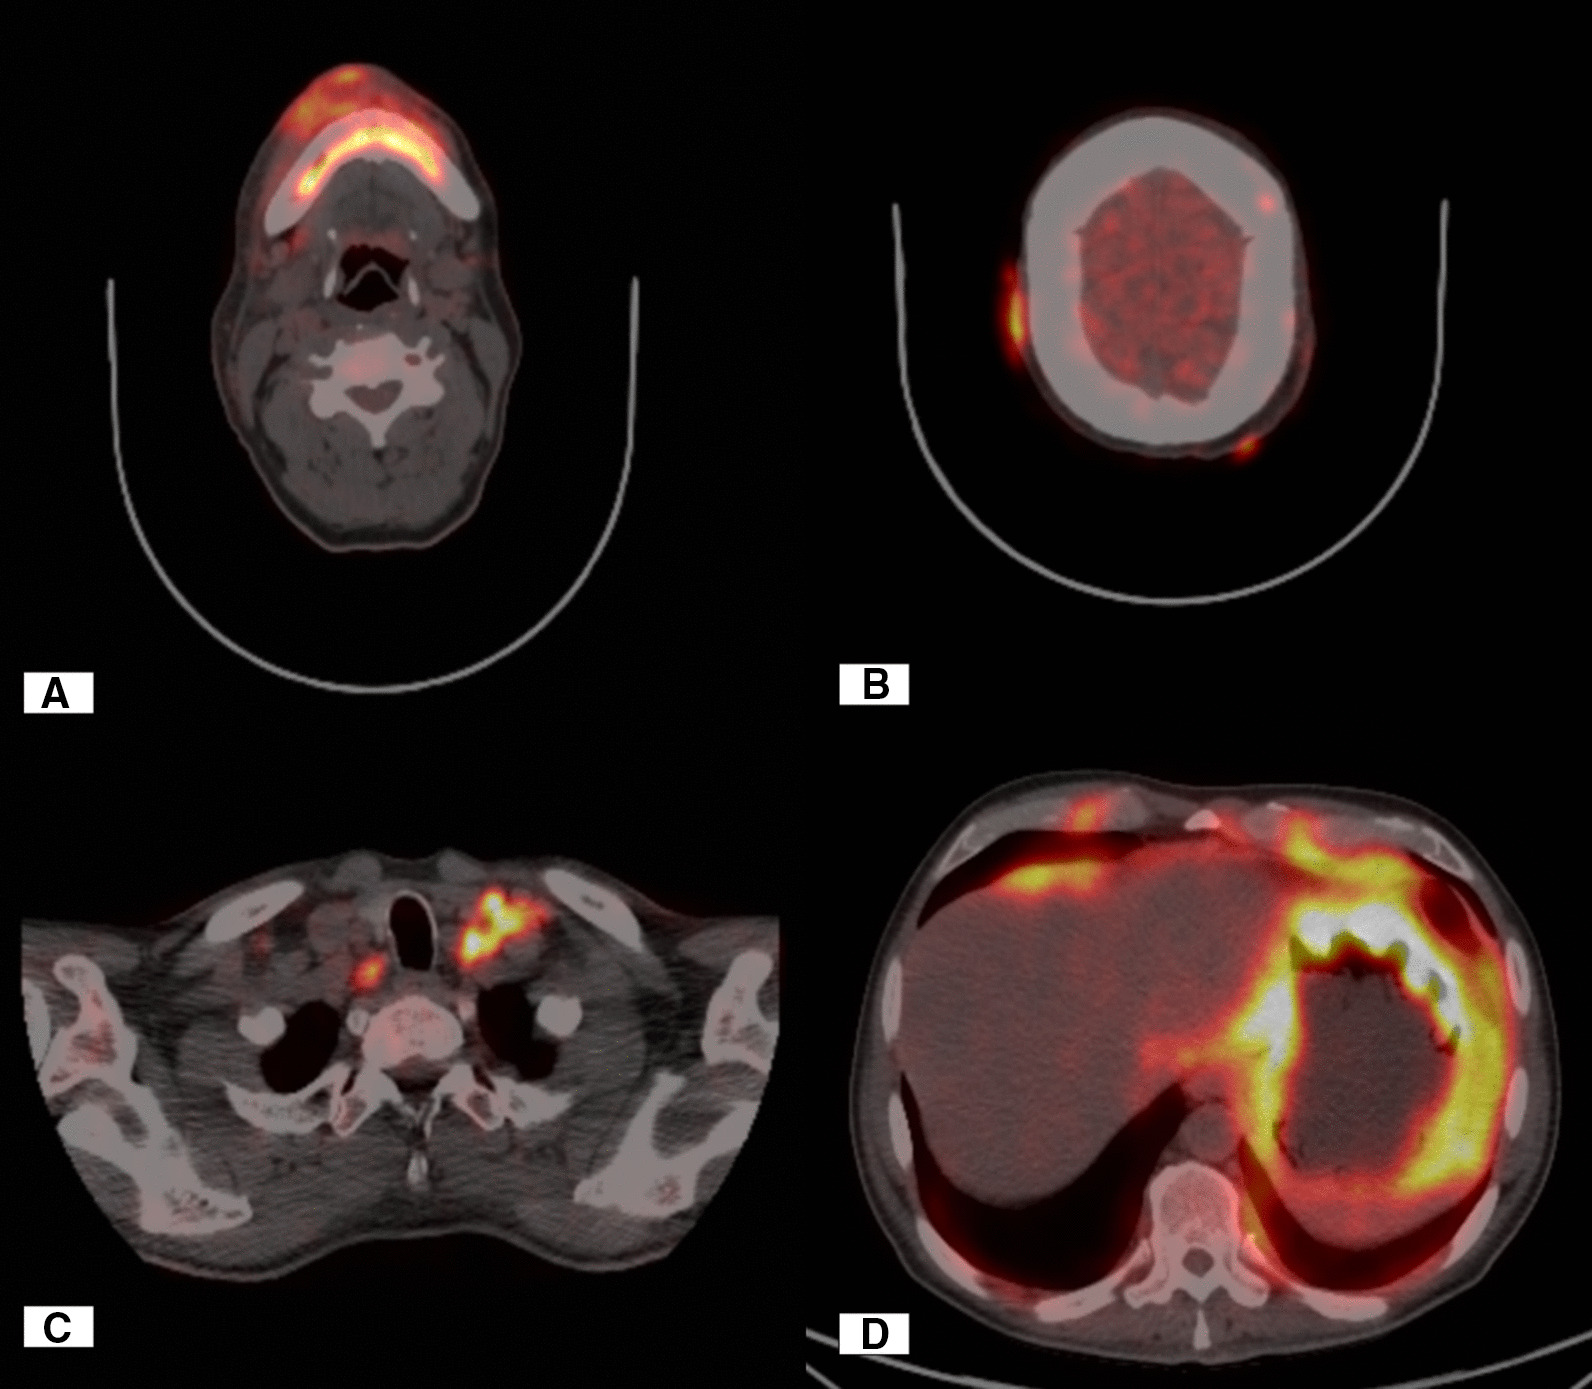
Images (18F-FDG-PET). A Hypermetabolic zone in soft tissues of the right lower jaw region, plus the ipsilateral submental nerve. B Hypermetabolic nodular lesions of subcutaneous location in the bilateral parietal region. C Hypermetabolic lesions suggestive of nodular cluster of the left internal jugular chain and right paratracheal adenopathy. D Axial image of abdomen with distended stomach. Thickening of the gastric wall is observed, which presents major metabolism throughout it
